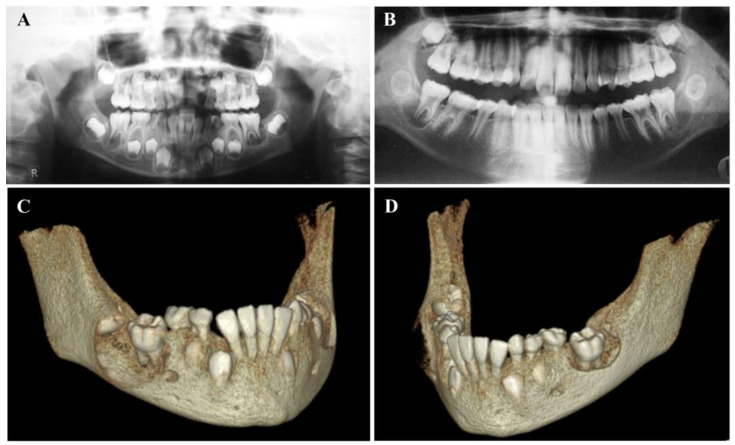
Panoramic radiographs and three-dimensional reconstructions in bone protocol of typical bilateral BBC lesions. (A). The panoramic radiograph displays distinct bilateral circular radiolucencies involving the erupting lower right first molar and lower left first molar. (Courtesy of Dr. Julio Corona-Rodriguez). (B). The panoramic radiograph shows bilateral radiolucencies associated with the lower left second molar and the lower right second molar. (Courtesy of Dr. Asher Ah-Tong Lim). (C,D) Three-dimensional reconstructions in bone protocol for the right (C) and left (D) mandibular regions highlight the lesions and their relationship with neighboring structures. (Courtesy of Dr. Cristhian Reynaldo Gomez Bautista).
